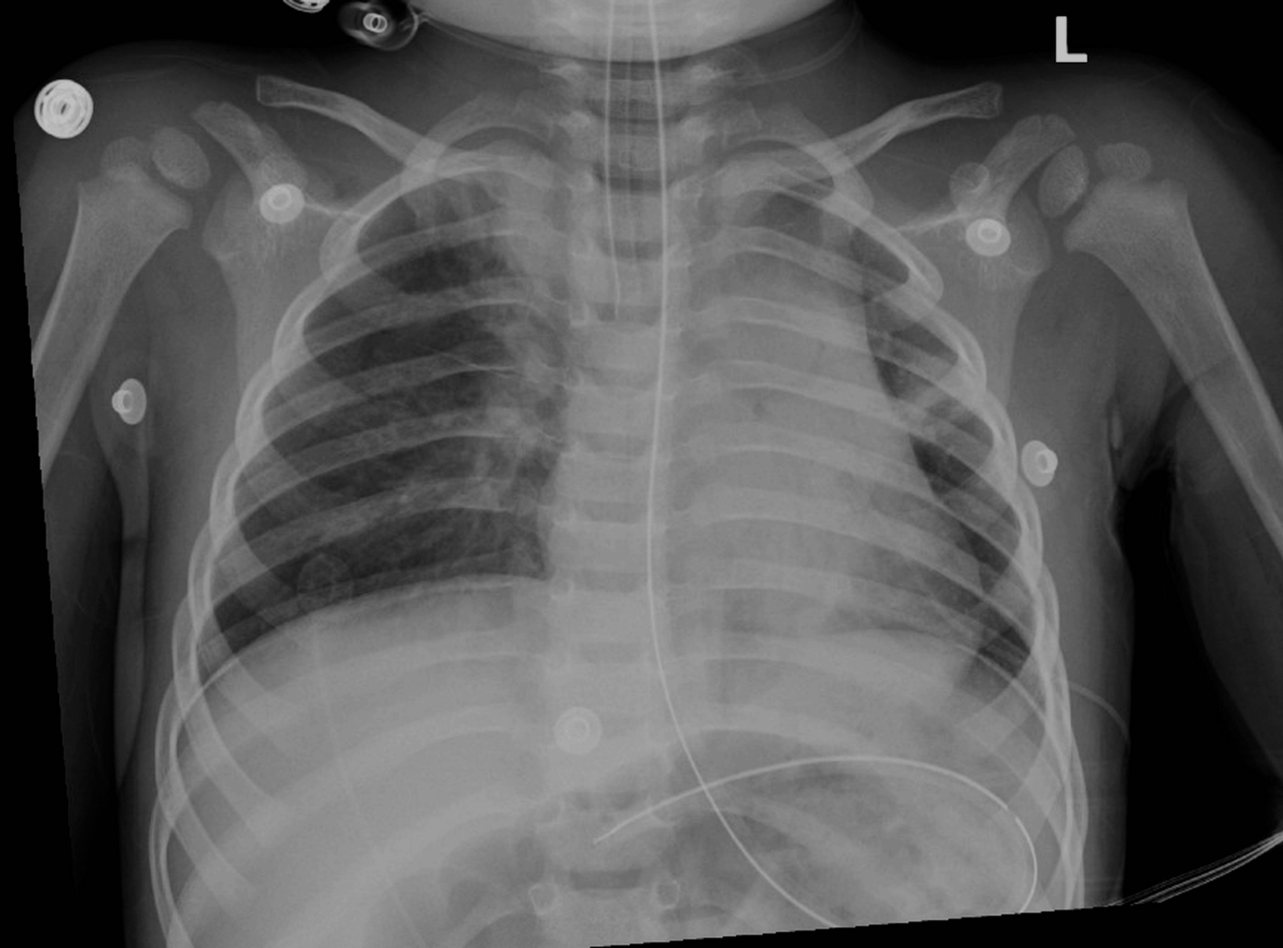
Chest radiograph obtained after intubation demonstrating no acute cardiopulmonary abnormalities.
